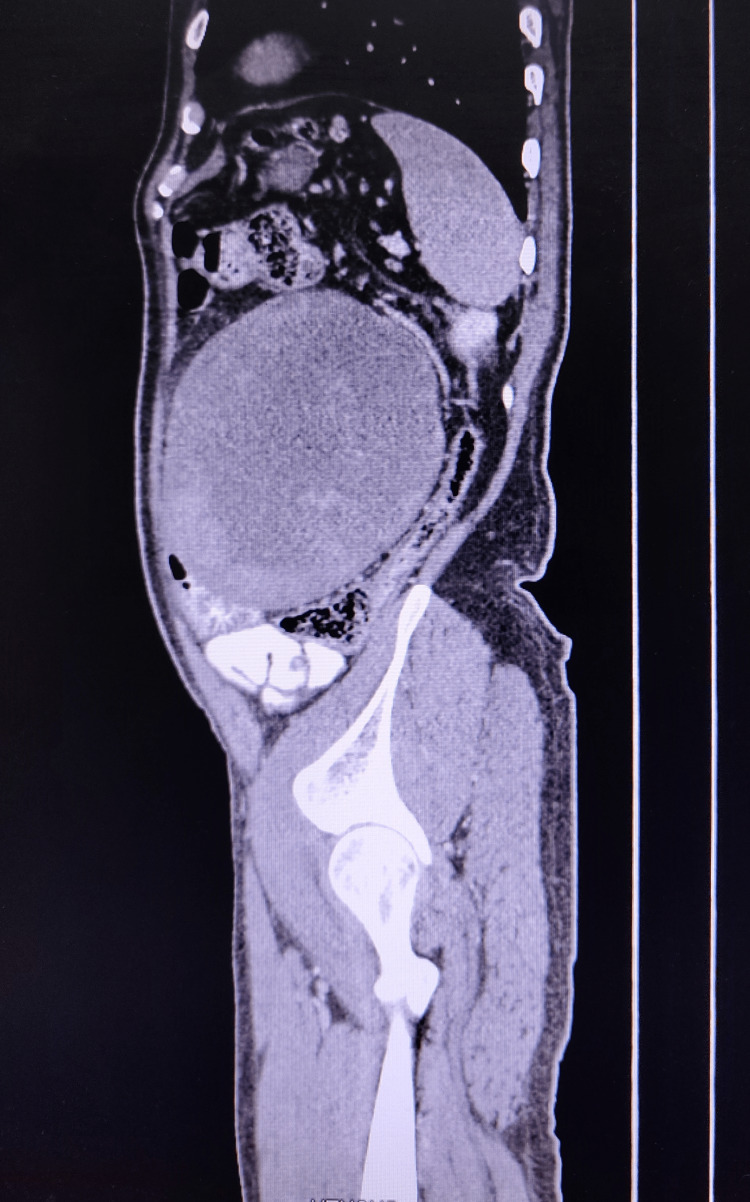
Sagittal section of CT abdomen showing the tumour and its relation with the surrounding structures
